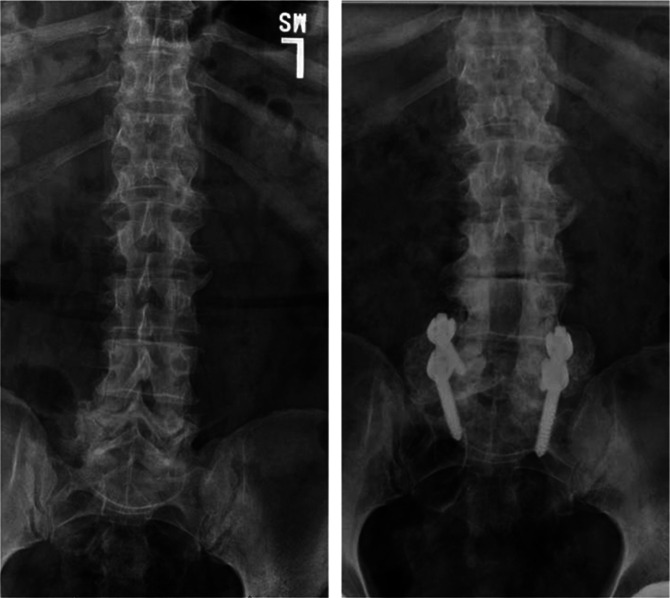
Preoperative and eight-year postoperative radiographs showing bony fusion of a patient who underwent posterolateral fusion with bone morphogenetic protein 2 (BMP-2) augmentation.
